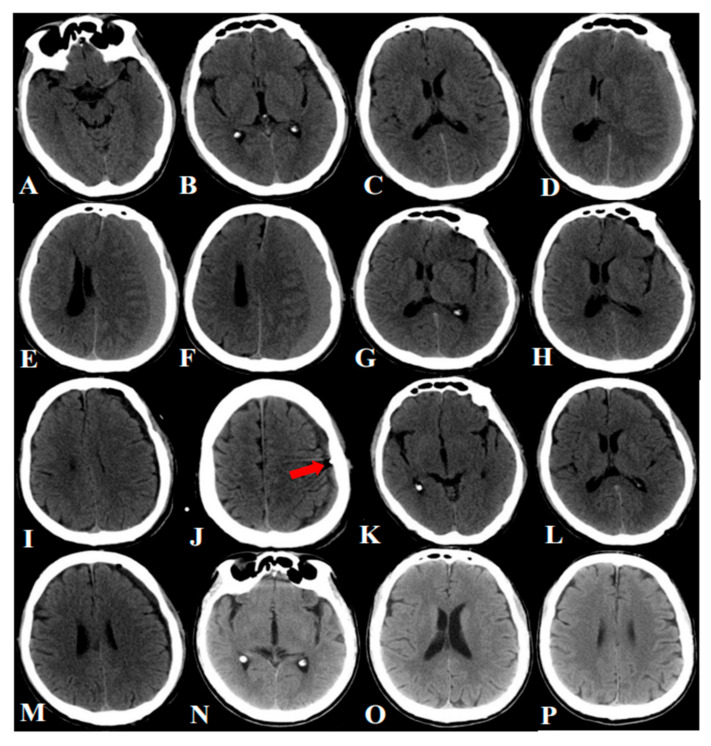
CT imagines of YL-1 puncture needle. (A–C) CT scanning after head trauma (three months before hospitalization). (D–F) CT scanning after admission. (G–J) CT scans one day before operation. (K–M) CT scanning at discharge. (N–P) Follow-up CT scanning (3 months).
